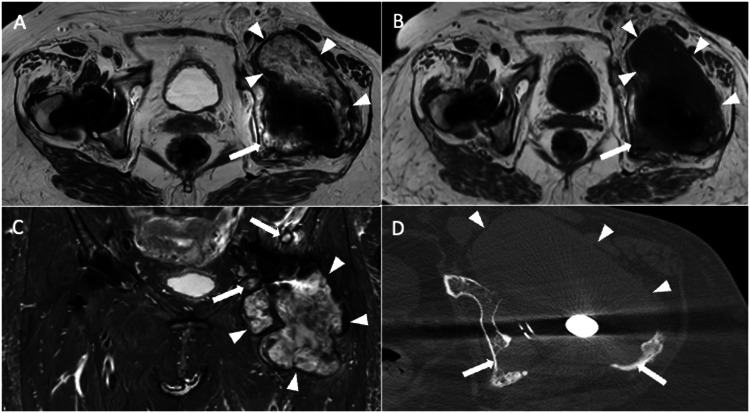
A 73-year-old female with bilateral THA, affected by particle disease on the left THA. Axial T2 weighted (A), axial T1 weighted (B), Coronal STIR (C), and axial bone window CT (D) images show high T2/low T1-signal collection with low-intensity rim (arrowheads), also visible on CT image, associated with periprosthetic osteolysis (arrows)
